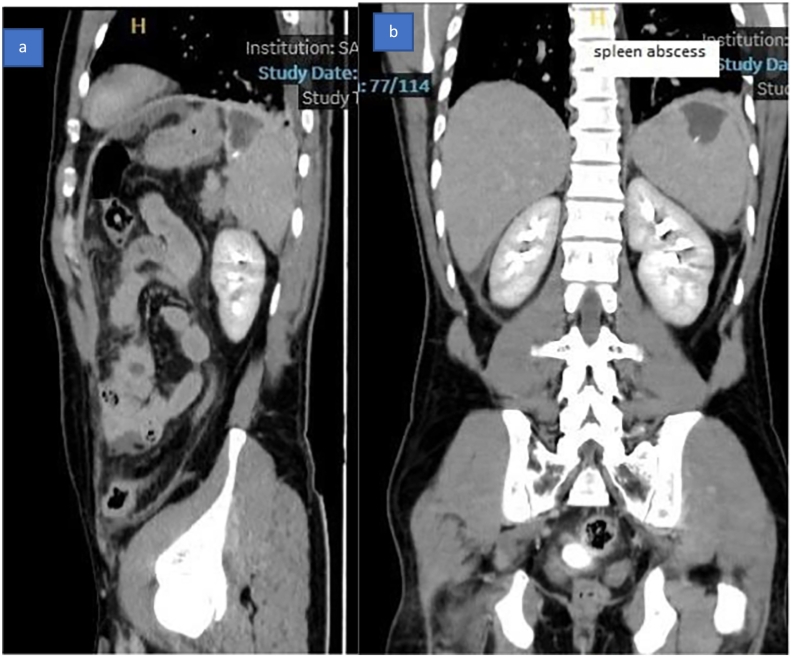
CT scan of the abdomen showing foreign body inside splenic abscess a: Sagittal view, b: Coronal view.
